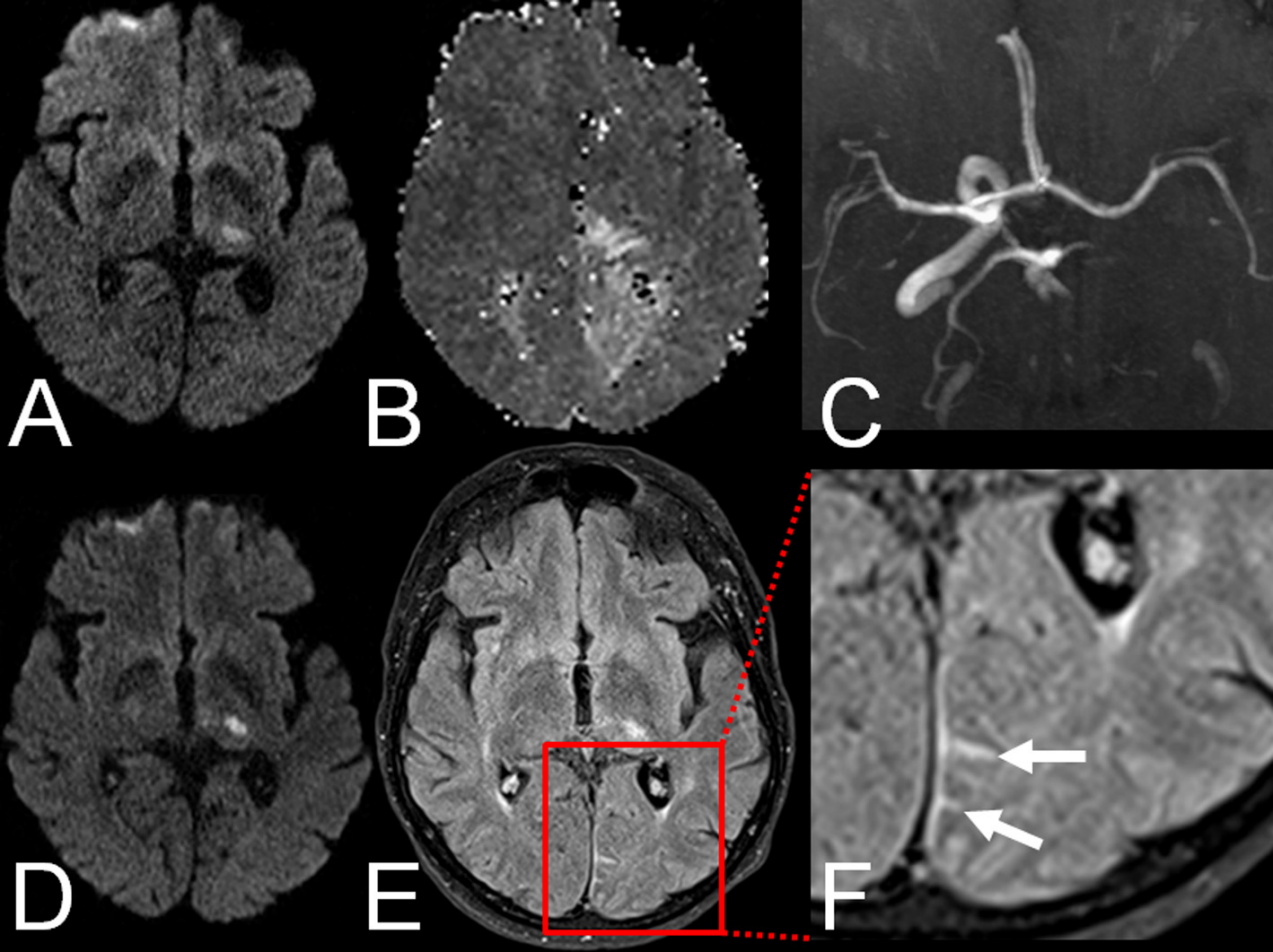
Example of HARM in a 67-year old patient with left posterior cerebral artery (PCA) infarction: A. Acute ischemic lesion in the left thalamus on DWI. B. Hypoperfusion in the left PCA territory on PWI. C. Proximal occlusion of the left PCA on TOF-MRA. D. Acute ischemic lesion in the left thalamus on follow-up DWI. E. HARM in the left PCA territory on FLAIR images. E. Magnification (1.5x) of HARM on FLAIR images (arrows).
